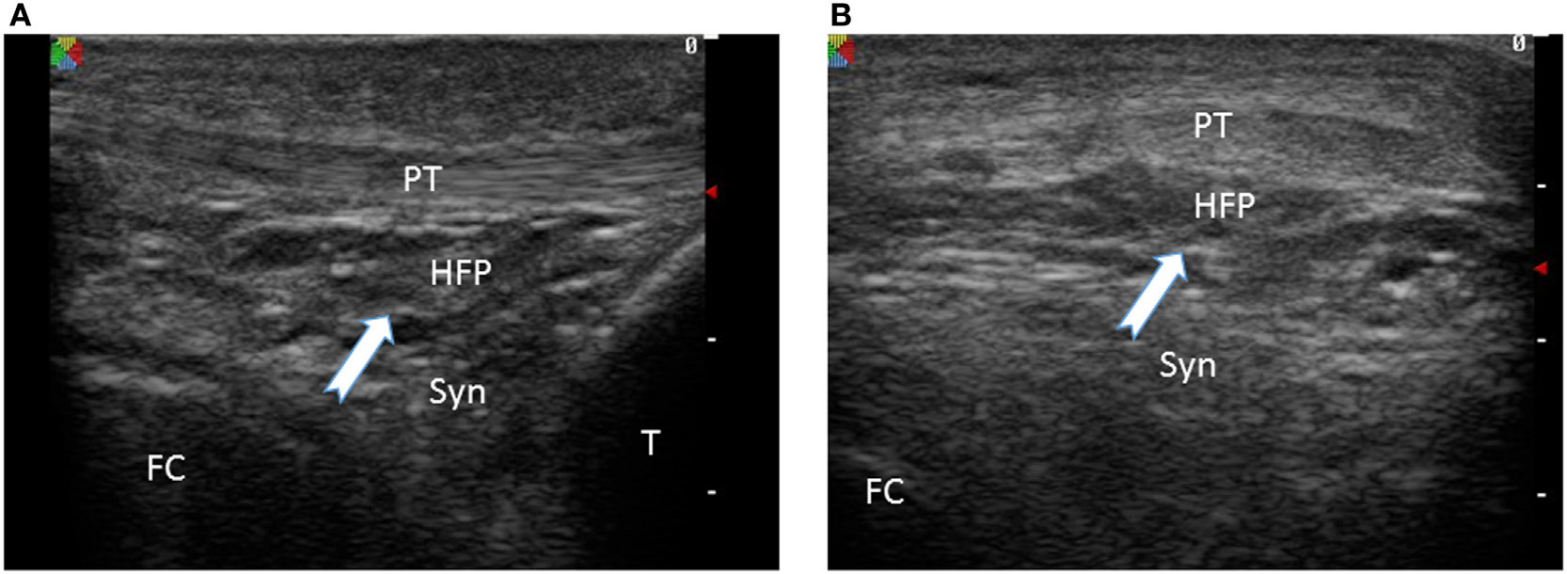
Ultrasound image of infrapatellar fat pad of a normal knee joint White arrow indicates the fat tissue with distinct ultrasound features compared to the underlying patellar tendon (PT) and synovial tissue (Syn). HFP, Hoffa fat pad; FC, femoral condyle; T, tibia. (A) Sagittal view, (B) coronal view.
